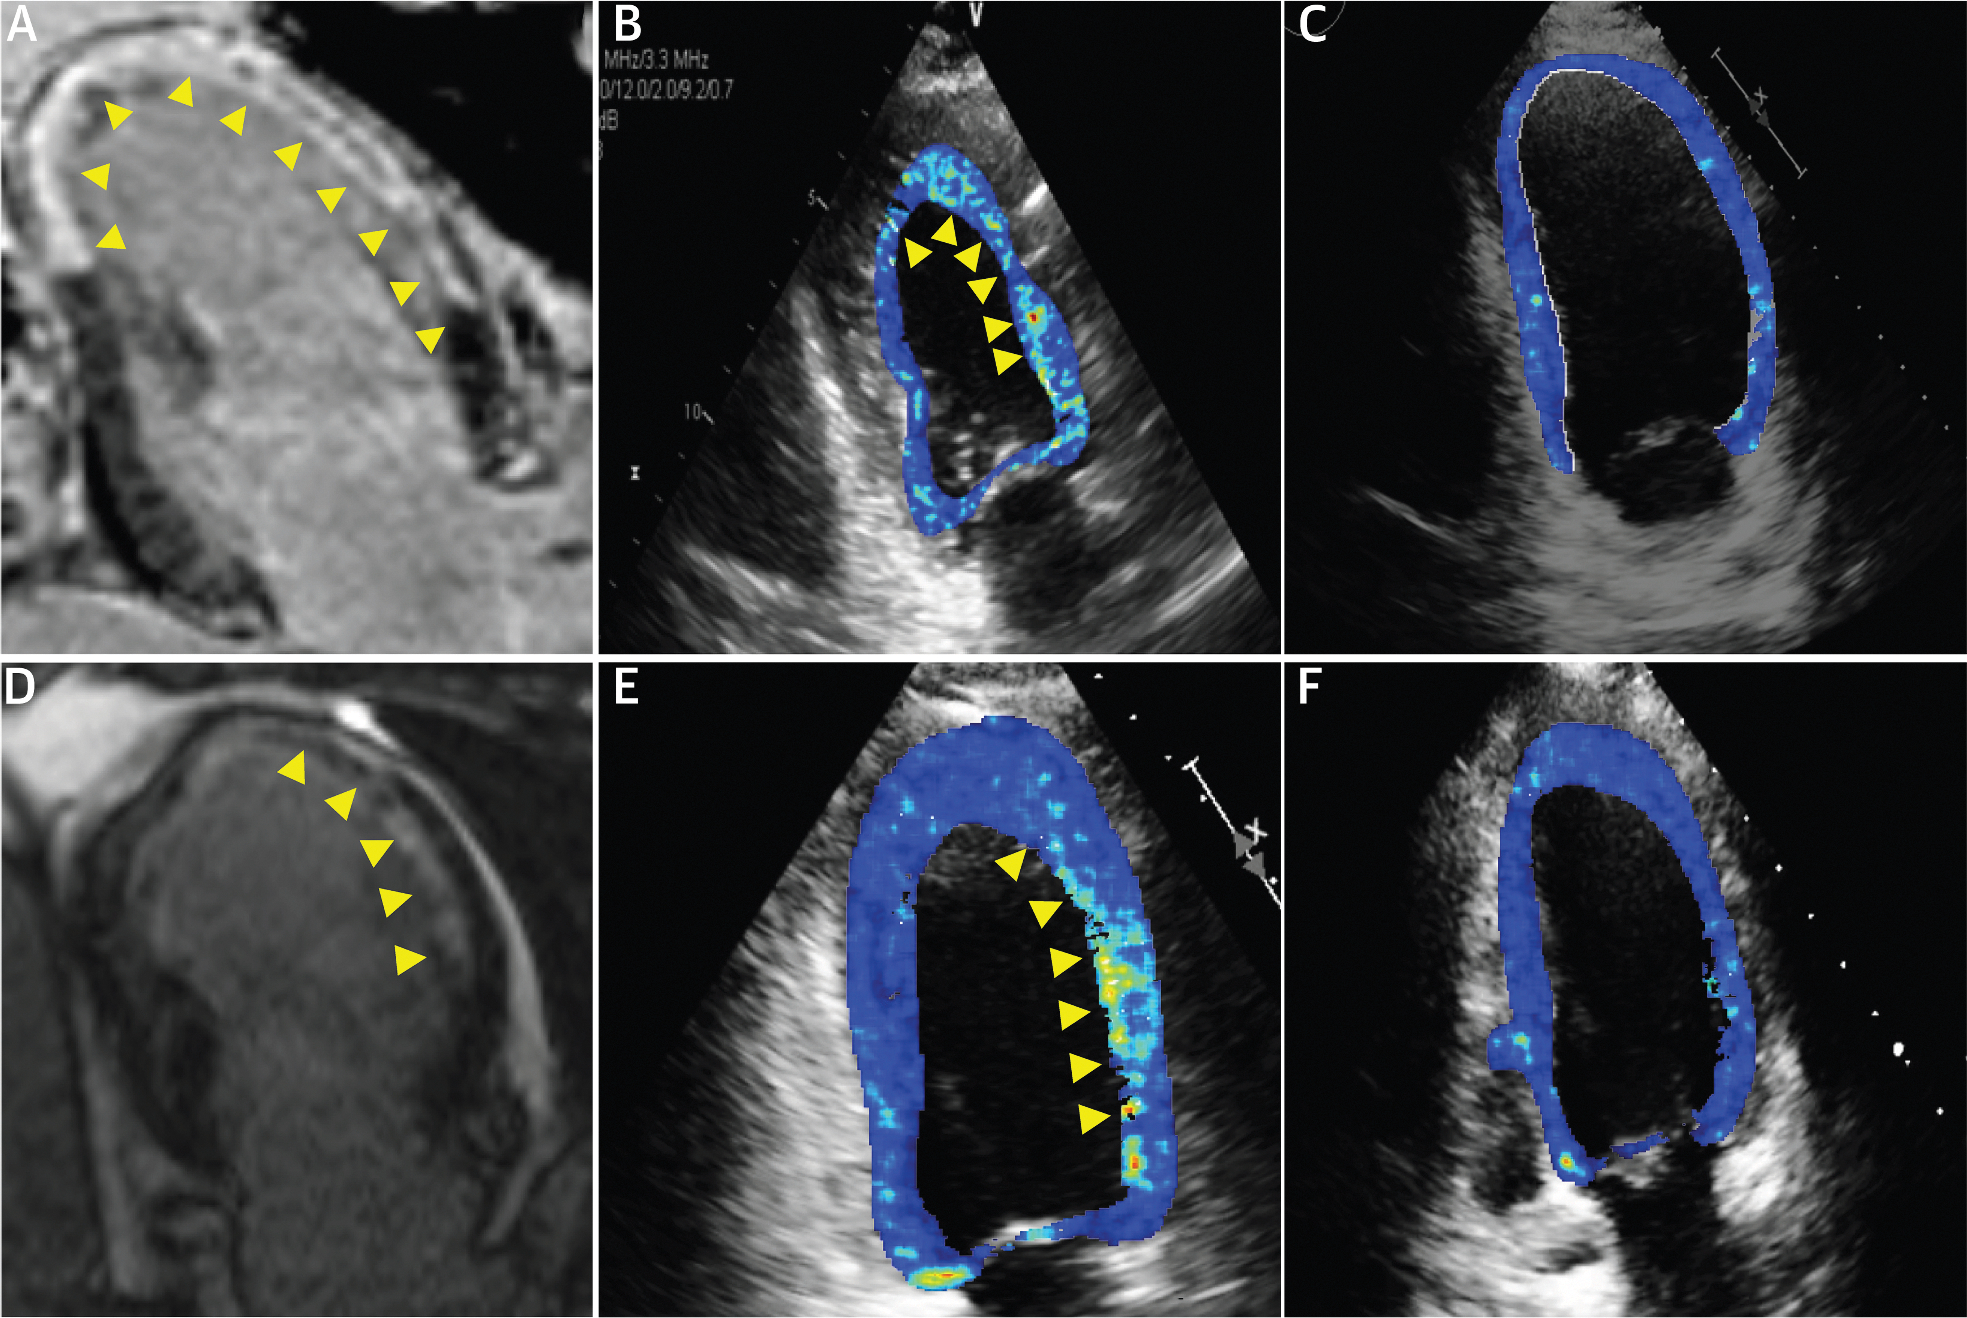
Ultrasomic Texture Feature Maps Over the Left Ventricular Myocardium Patient 1 (A, B) and patient 2 (D, E): paired cardiac magnetic resonance (CMR) and 2-chamber echocardiographic views. (A, D) Arrowheads indicate infarcted myocardium on late gadolinium enhanced images. (B, E) Arrowheads indicate the same regions as identified by the corresponding radiomics feature. (C, F) Control subjects without infarction.
